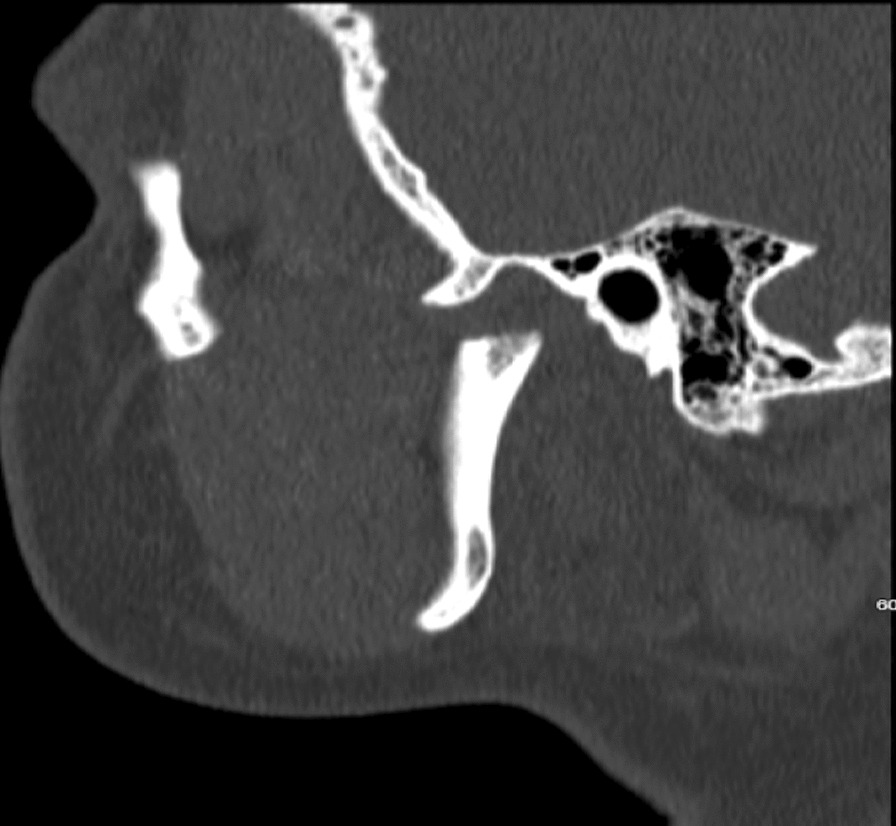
Computed tomography maxillofacial sagittal view demonstrating fracture of neck of right mandible
